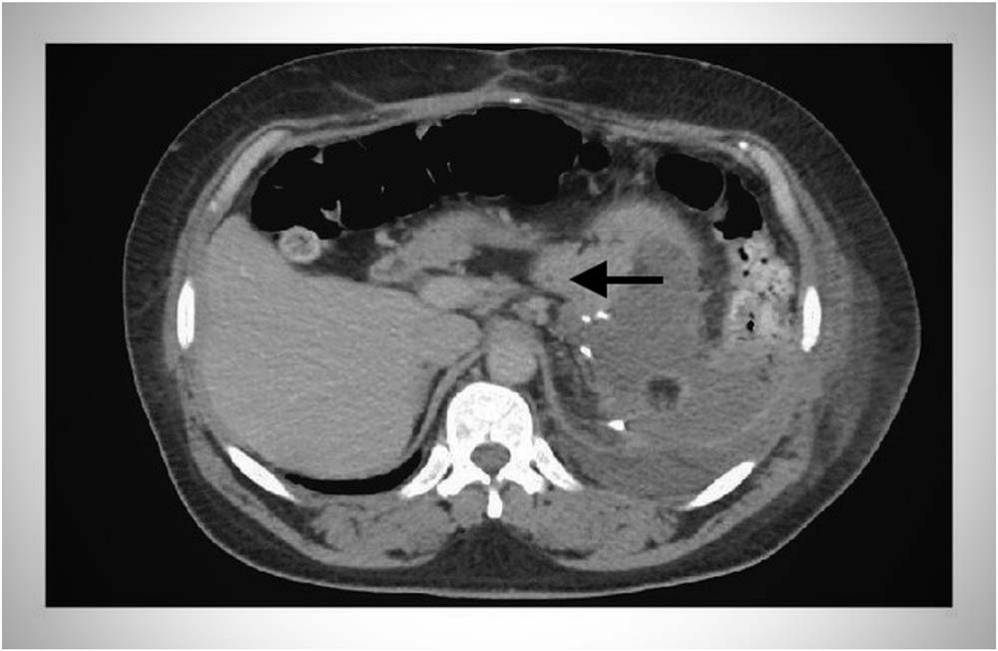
CT scan of abdomen showing fluid collection around the tail of the pancreas (black headed arrow).
